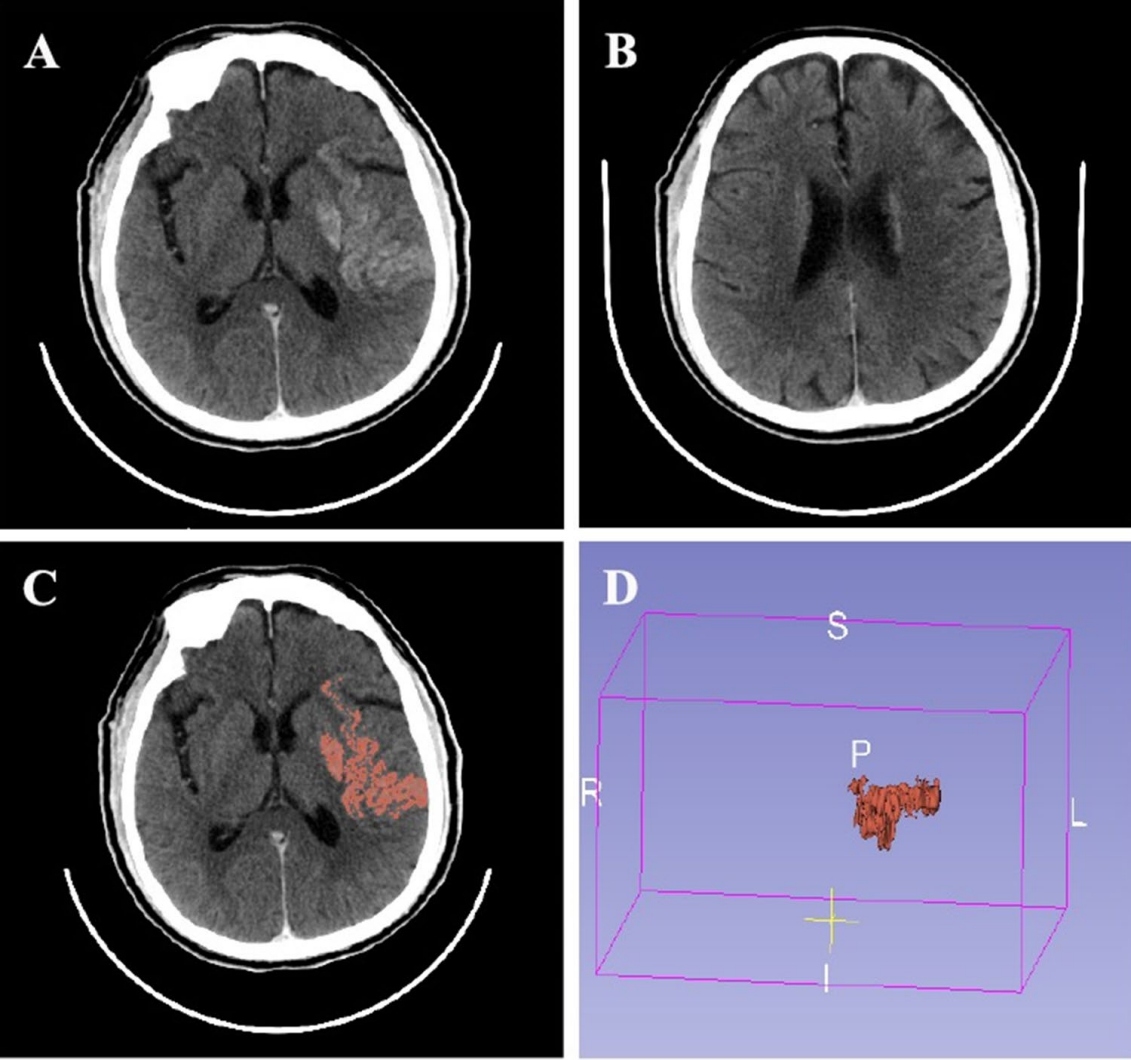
A 71-year-old patient presented with left-sided weakness (CE-ASPECT 6 and CE-Volume 9.041 ml). (A) and (B) Showing CE in the insula, lenticular nucleus, M1 and M2 ASPECTS regions. Thus, the CE-ASPECTS was counted as 6. (C) and (D) The three-dimensional structure of CE in the brain was reconstructed with a volume of 9.041 ml.
